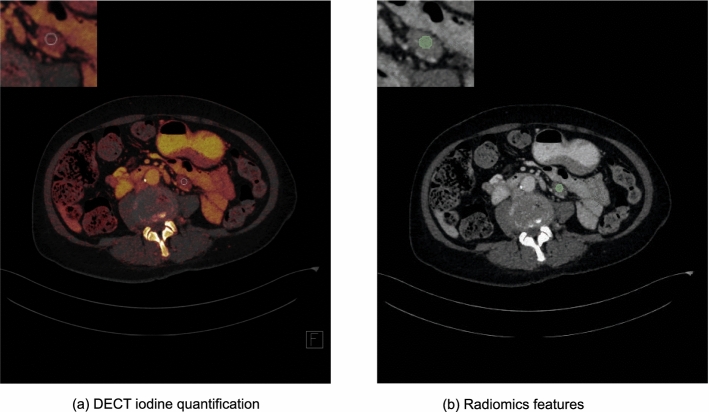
DECT iodine quantification and radiomics feature segmentation. A 66-year-old female patient with diffuse large B-cell lymphoma (DLBCL). Diagnosis was confirmed by lymphadenectomy and PET/CT. a Axial DECT-based iodine map image with region-of-interest (ROI) measurement of the respective lymph node. b Axial DECT image with standard volume-of-interest (VOI) measurement of the respective lymph node for radiomics feature extraction
